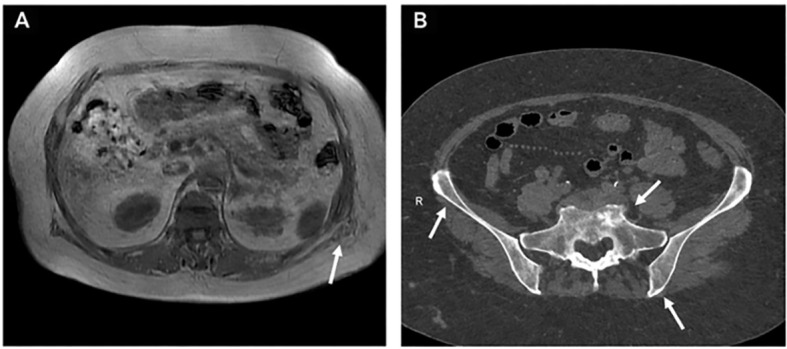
Radiological imaging of skeletal involvement in Erdheim–Chester disease (ECD). (A) Sagittal T1-weighted magnetic resonance imaging (MRI) of the lumbar spine showing bilateral cortical sclerosis and abnormal vertebral signal changes characteristic of ECD. (B) Contrast-enhanced computed tomography (CT) of the pelvis demonstrating cortical sclerosis, irregular bone remodeling, and cortical thickening, which are typical skeletal features of ECD. This figure highlights the prominent osseous changes observed in ECD, including cortical sclerosis and irregular bone remodeling, which serve as important diagnostic clues.
